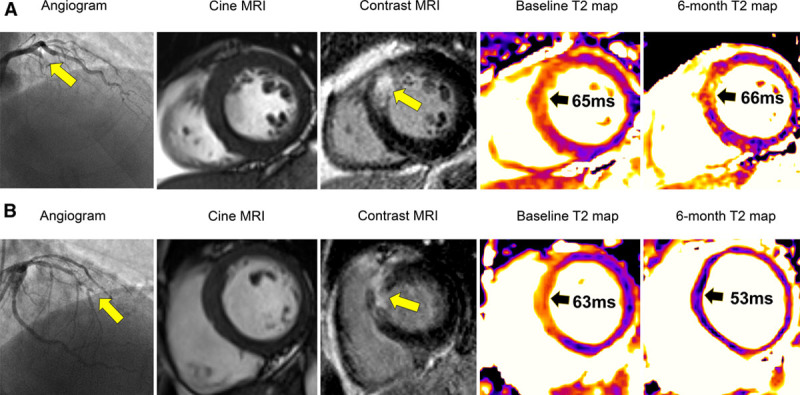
Two patients with a similar presentation of acute anterior ST-segment–elevation myocardial infarction. Both patients were treated by percutaneous coronary intervention and with the same antithrombotic drugs. At the end of the procedure, both patients had Thrombus in Myocardial Infarction (TIMI) coronary flow grade 3 in the culprit left anterior descending artery. A, A patient with persistent infarct zone T2 hyperintensity: cardiac magnetic resonance (CMR) imaging was performed 2 days post-revascularization. T2 mapping revealed an infarct zone T2 value of 65 ms. CMR performed at 6 mo revealed a persistently high infarct zone T2 value of 66 ms in a matched myocardial slice position to baseline. Left ventricular (LV) end-diastolic volume increased from 143 to 175 mL at 6 mo representing adverse remodeling. This patient was readmitted with heart failure after the 6-mo CMR scan. B, A patient without persistent infarct zone T2 hyperintensity: CMR was performed 2 days post-revascularization. T2 mapping revealed an infarct zone T2 value of 63 ms. CMR performed at 6 mo revealed a lower infarct zone T2 value of 53 ms. LV end-diastolic volume decreased from 120 to 118 mL at 6 mo. This patient had an uncomplicated clinical course. MRI indicates magnetic resonance imaging.
